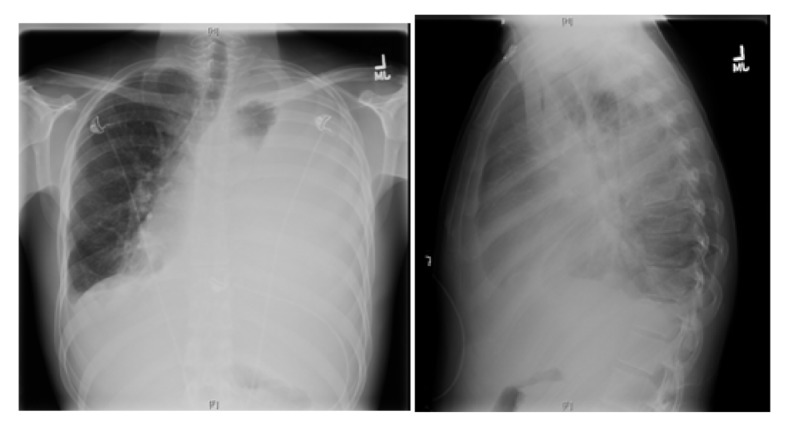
X-ray chest showing near complete opacification of left lung due to massive pleural effusion with shift of mediastinum to right.
